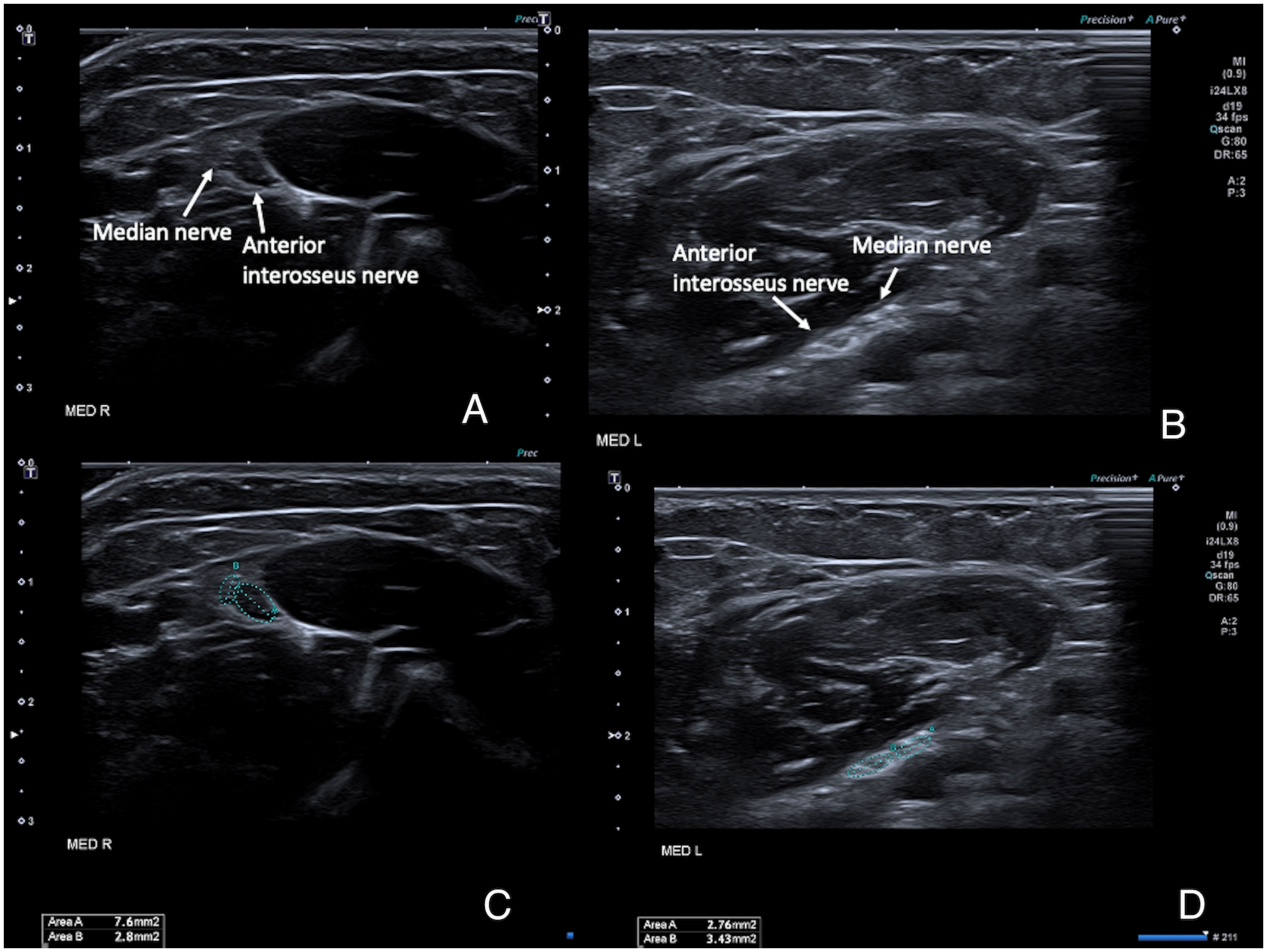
High-resolution ultrasound images of the right (A,B) and left (C,D) median nerves at the level of the elbow, with the nerves running between the deep and superficial head of the pronator teres muscle. (A,C) show the anatomical configuration, with an unusually small median nerve proper and a comparatively larger anterior interosseus nerve, and even more pronounced on the right (where a thumb was present) which is the opposite of normal. (B,D) show the same images with the cross-sectional area measurements superimposed. Normal adult average reference cross-sectional areas (CSAs) in this region is around 7–9 mm^2^ for the median nerve proper at the elbow level and 3–4 mm^2^ for the anterior interosseus nerve.
